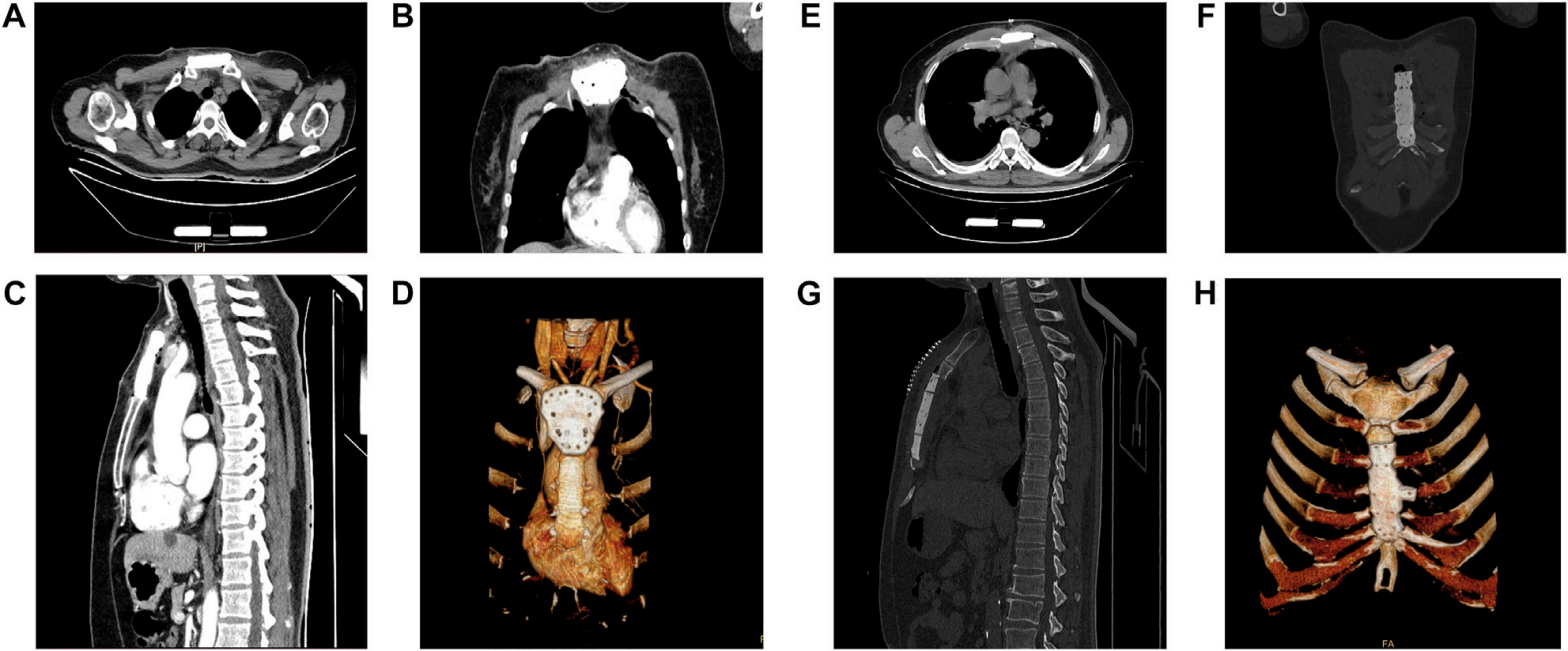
Postoperative computerized tomography (CT) images of the patients. (A,E) The CT images of sternal prosthesis in the transverse view.(B,F) The CT images of sternal prosthesis in the coronal view. (C,G) The CT images of sternal prosthesis in the sagittal view. (D,H) The 3D reconstruction of the sternal prosthesis on CT is demonstrated.
